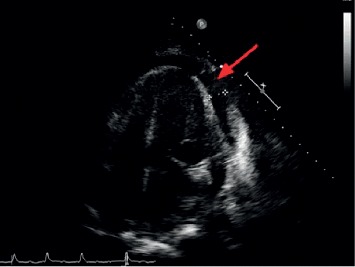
Transthoracic echocardiogram (TTE) demonstrating a large pericardial effusion (red arrow).
